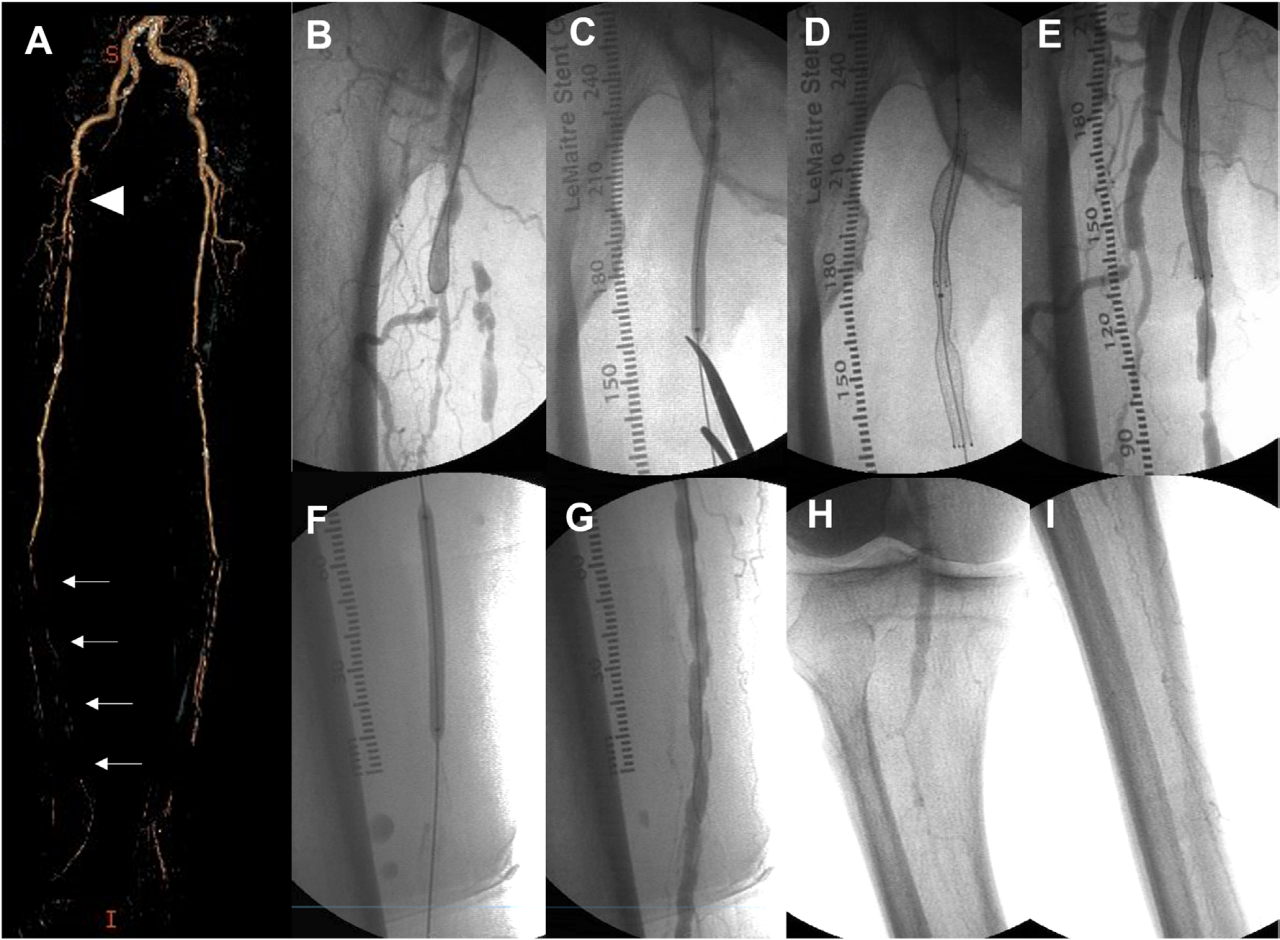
Fig. 1 Findings of revascularization. Preoperative computed tomography angiography showing diseased right superficial femoral artery (SFA, arrowhead) and chronic total occlusion (CTO) of the infrapopliteal arteries (arrows) (A). Additionally, the angiography demonstrates CTO of a proximal segment of the right SFA (B). A guidewire was crossed into the CTO lesions by endarterectomy of the distal SFA, and stents were placed after balloon angioplasty was performed (C, D). A distal segment of the right SFA was stenosed (E), and balloon angioplasty was performed (F, G). Infrapopliteal arteries were diseased; however, a contrast equipment was poor to show these segments (H, I).
